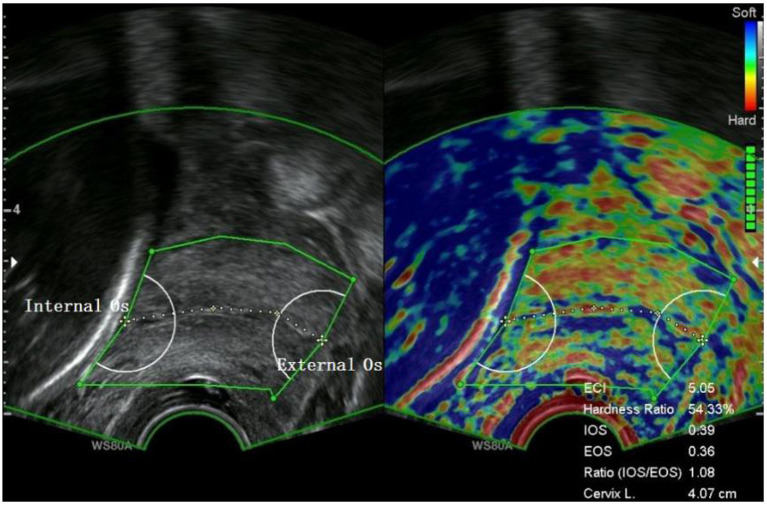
Transvaginal ultrasound elastography of the cervix showing measurement of elasticity parameters and cervical length.
